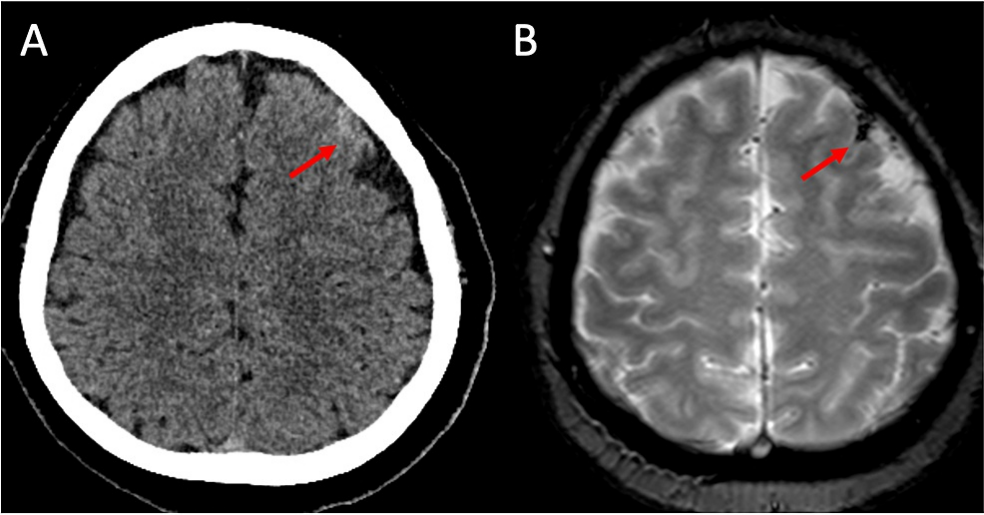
Convexal subarachnoid hemorrhage Brain non-contrast computed tomography at seven years of disease showed scarce hyperdense blood content in the upper left frontal sulcus (A), which correlates with hemosiderin deposition in the T2-weighted gradient-echo MRI (B).
